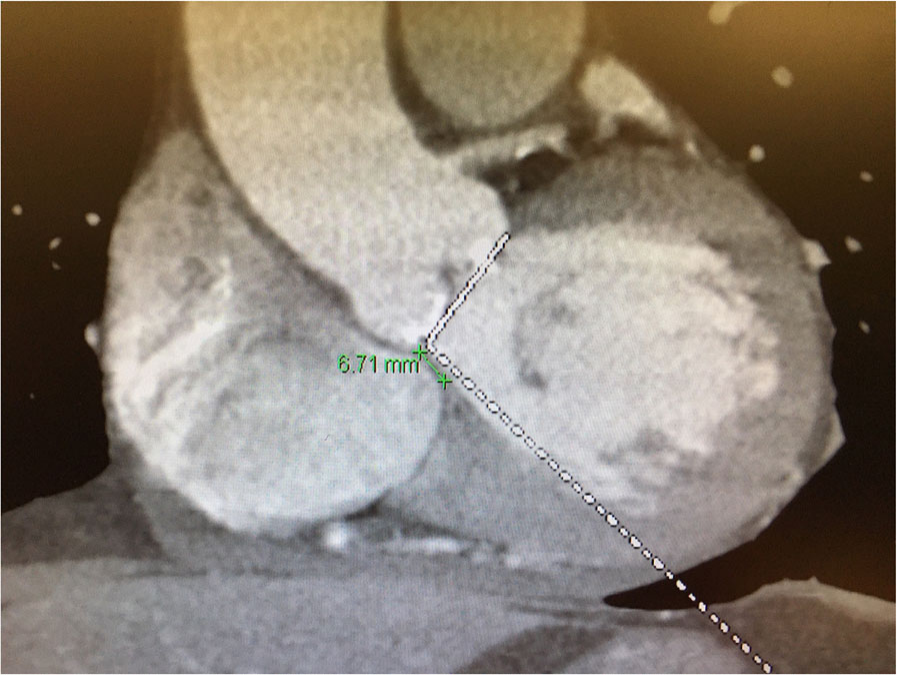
Measurement of infra-annular membranous septal length (IMS) on coronal cut computed tomography images. Distance measured between lowest point of annulus and start of muscular interventricular septum (Green line with measured dimension of IMS length)
